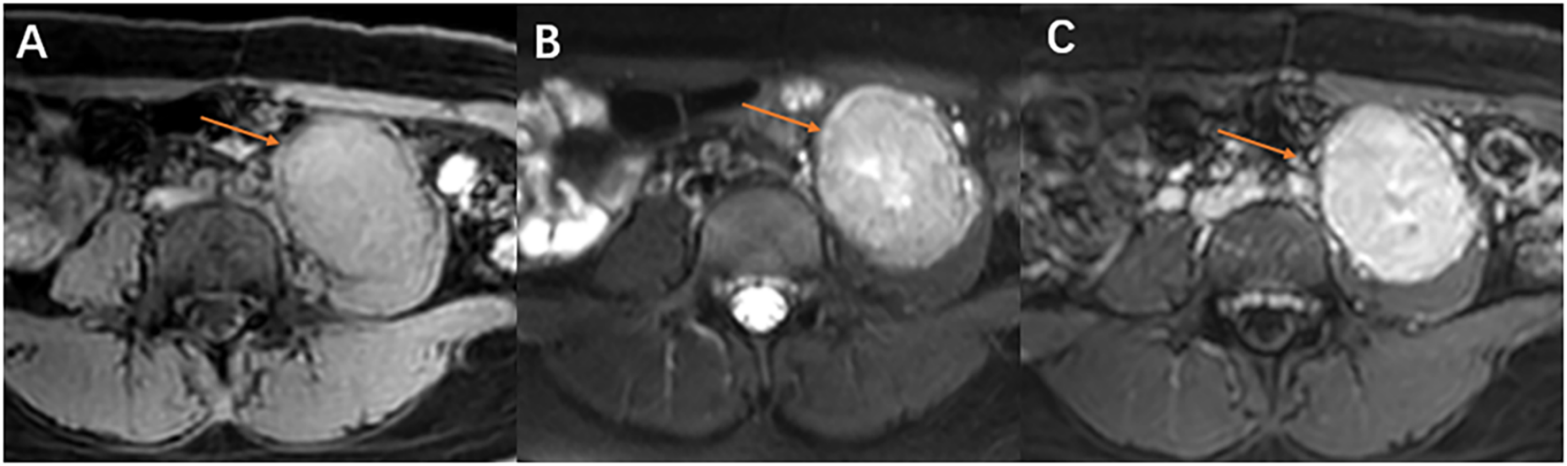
MRI, Magnetic resonance imaging reveals the tumor in the left psoas major muscle (red arrow). (A) On T1WI, the mass in the left psoas major, with a round-like appearance, demonstrated an isosignal. (B) On T2WI, the mass exhibited a high signal, with a slightly elevated signal visible in the center. Tiny blood vessels are visible at the edge. (C) The MRI enhancement scan indicates a notable blood-rich enhancement in the tumor of the left psoas major muscle.
